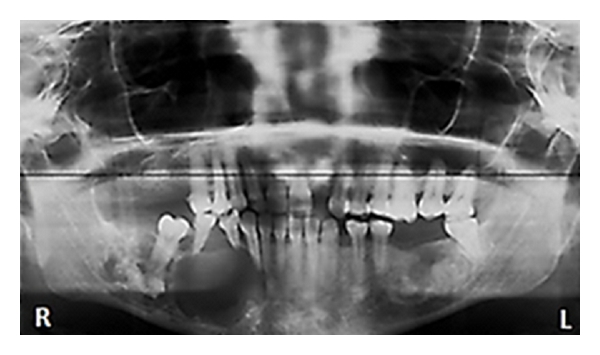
An orthopantomogram of the patient in case 2 showing a significant bone destruction of the right mandible extending from the lower right lateral incisor to a region just short of the mandibular angle. The radiolucency also encompasses the irregular mass of radio-opacity just apical to right mandibular first molar. The radiograph shows a similar irregularly shaped radio-opacity within the body of the left mandible.
